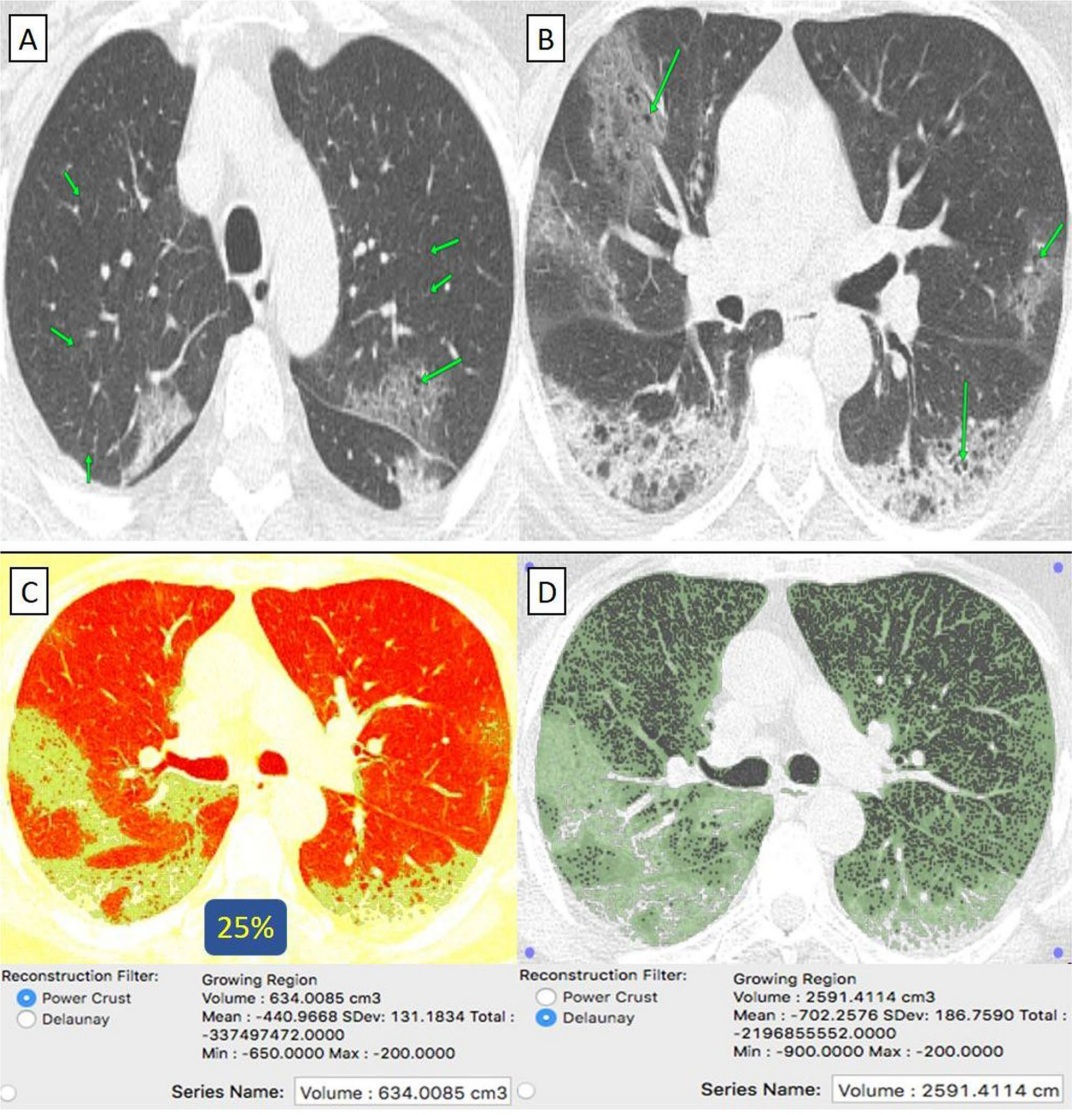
A 75-year-old male patient with history of lung emphysema and had received “Phizer-Biontech” vaccine then got COVID-19 infection. A, B Axial chest CT cuts (lung window) showed bilateral centrilobular emphysema as well as peripheral located and to lesser extent bronchocentric ground-glass pneumonic patches with cystic changes. C CT-Volumetry of the pathological lung parts averages 634 cc. D CT-Volumetry of total lung volume averages 2591 cc. So, 25% of the lung parenchyma was diseased (Score 1)
